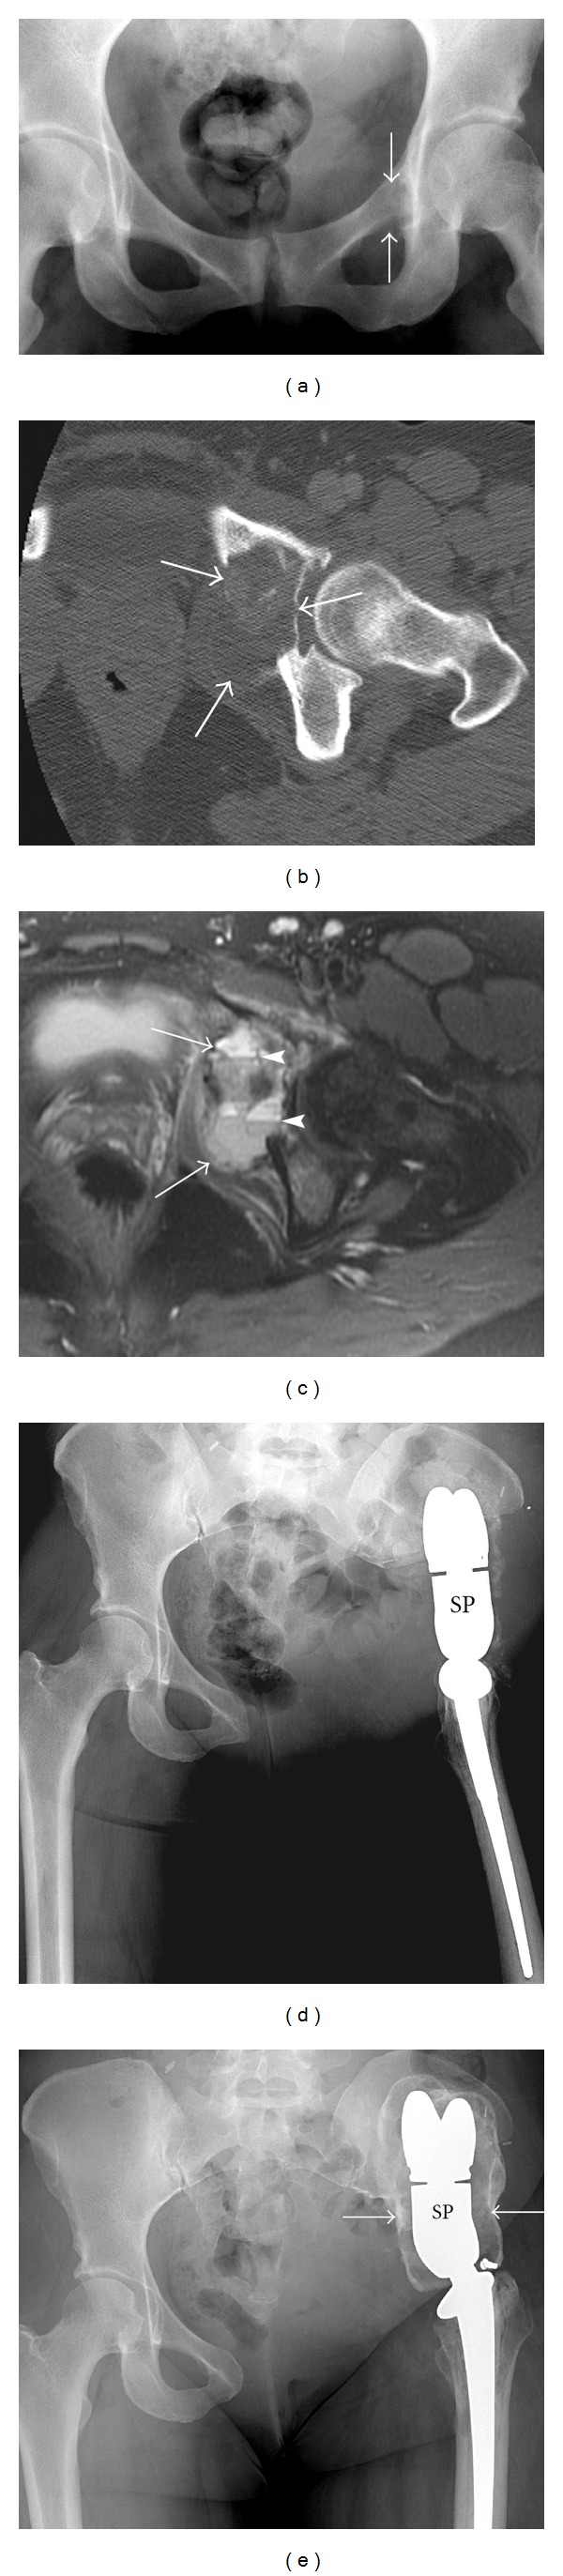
Osteolytic osteosarcoma. A 35-year-old female presenting with left hip pain diagnosed with aggressive lytic osteosarcoma. AP radiograph (a) demonstrates a subtle lytic lesion (arrows) in the left superior pubic ramus extending to involve the medial acetabulum. The extent of this predominantly lytic lesion with pockets of dense osteoid matrix is better appreciated on axial CT (arrows) (b). Axial T2 fat-saturated image (c) of the same lesion (arrows) depicts multiple fluid-fluid levels (arrowheads). The patient underwent left hemipelvectomy with placement of saddle prosthesis (SP) (d). A shell of heterotrophic ossification formed around the prosthesis (arrows) (e) is an expected finding, which helps stabilize the prosthesis and should not be misinterpreted as recurrence.
